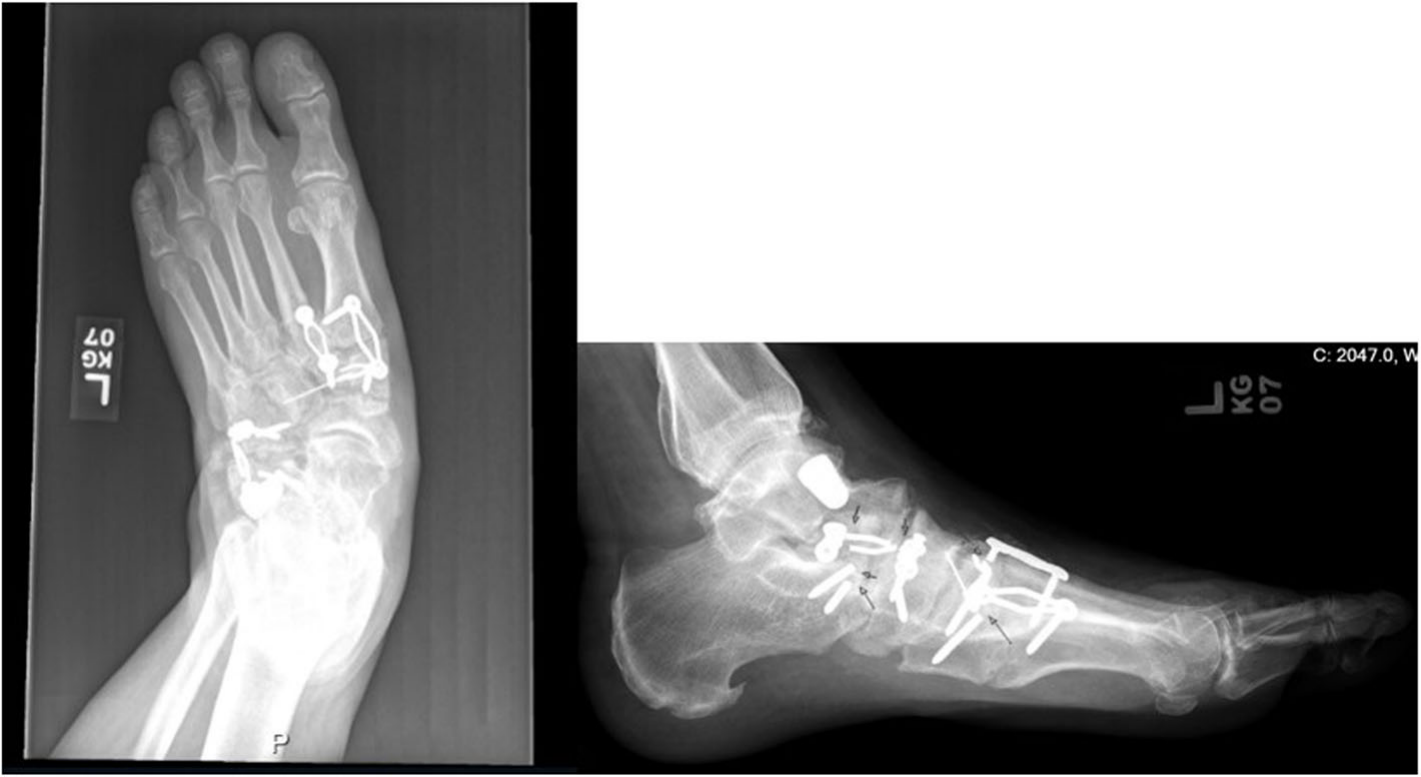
preoperative Radiographs showing failure of the fixation construct and the patient developed Charcot arthropathy of the midfoot
